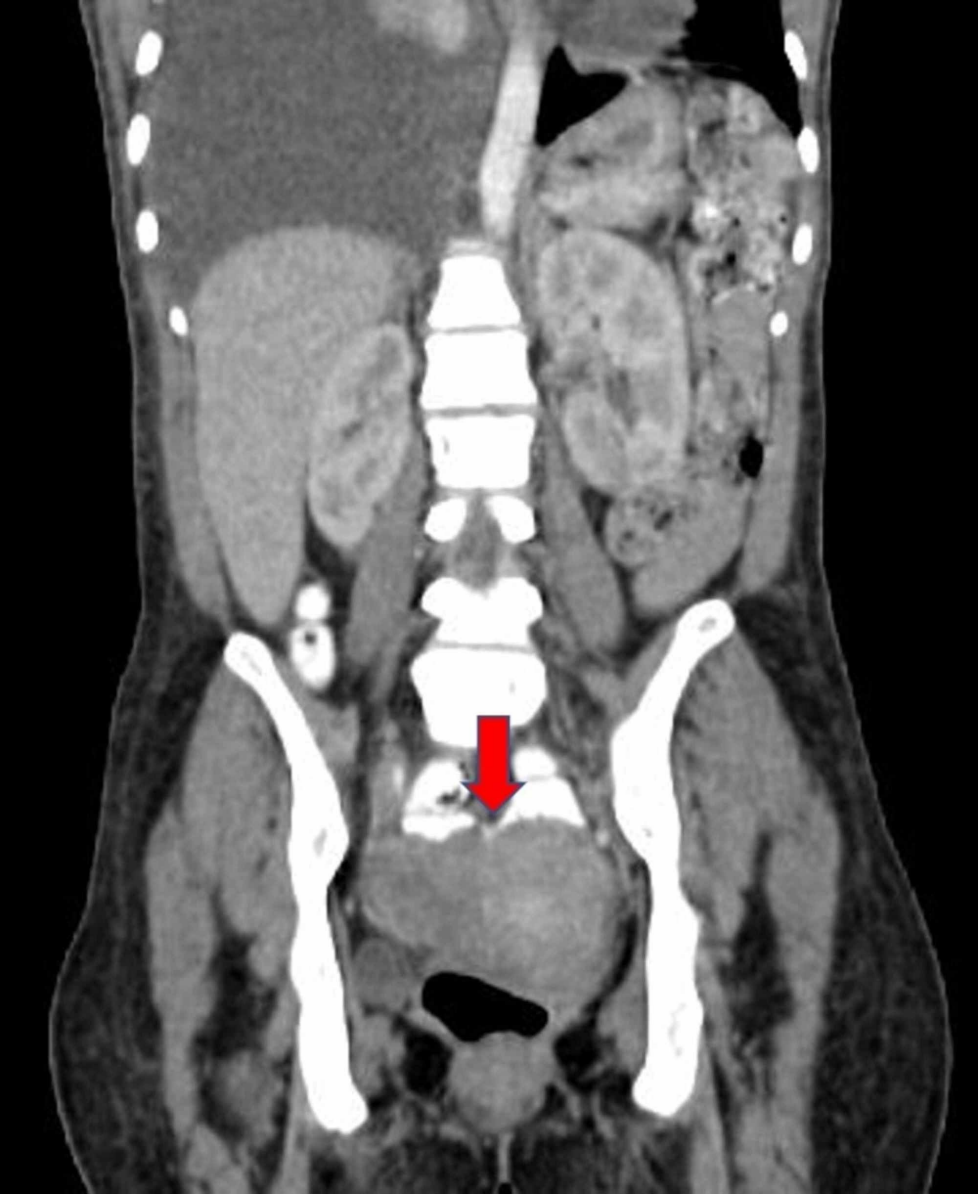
Coronal view of the abdomen and pelvis CT demonstrating a heterogeneous partially cystic right adnexal lesion, six months after starting alectinib.
